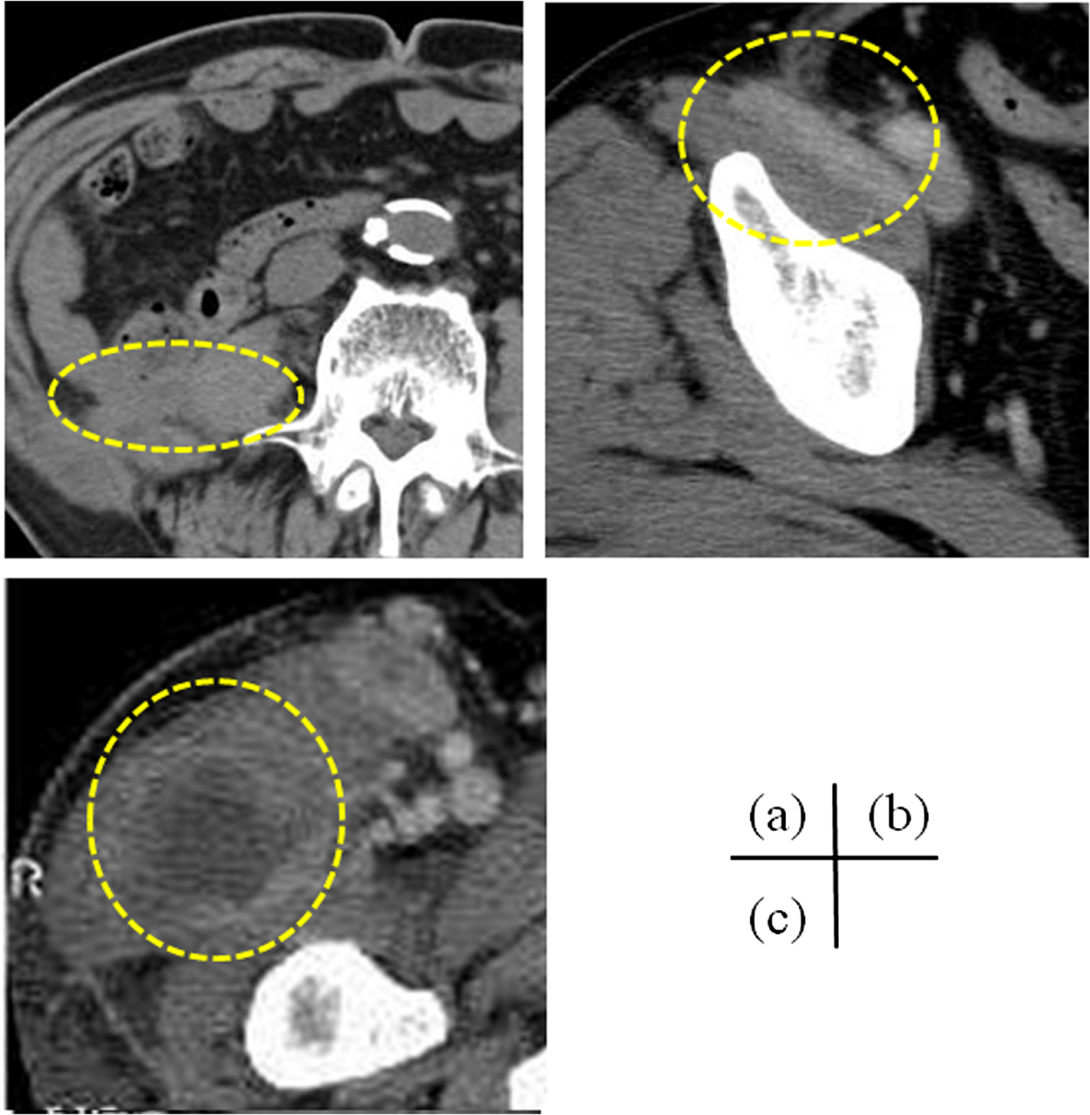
a–c A computed tomography scan reveals a retroperitoneal abscess 2 months after the surgery for retroperitoneal granulation and abscess. It also shows a fistula extending from the psoas abscess to the femoral abscess, as in Fig. 1 (*encircled by the dotted line*)
